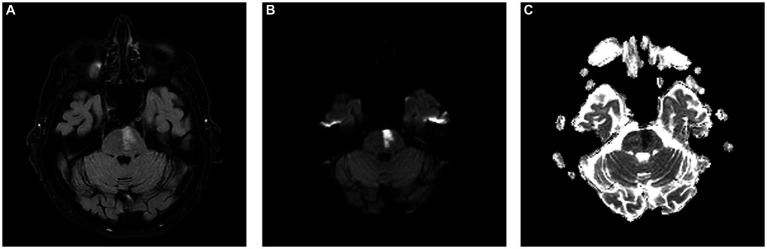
Brain MRI. Acute left pontine ischemia. (A) FLAIR transverse image, (B) DWI transverse image, (C) ADC transverse image.
